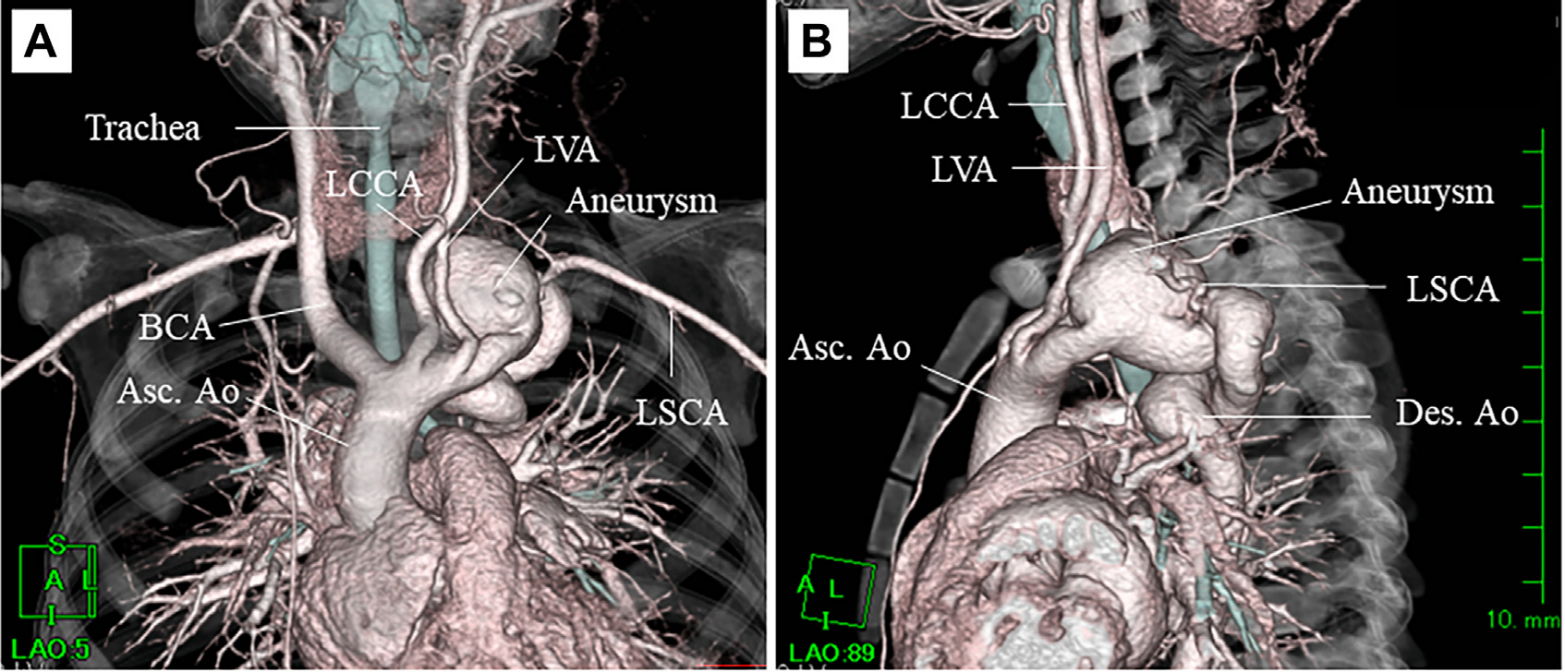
Preoperative 3-dimensional computed tomography revealed a tortuous aortic arch aneurysm from the distal aortic arch to the descending aorta (Des. Ao). (A) Frontal view. (B) Left lateral view. (Asc. Ao, ascending aorta; BCA, brachiocephalic artery; LCCA, left common carotid artery; LSCA, left subclavian artery; LVA, left vertebral artery.)
